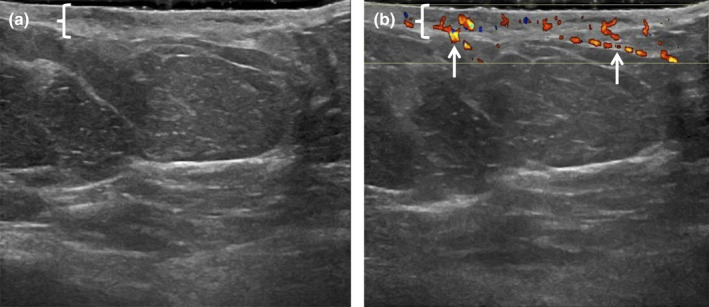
30‐year‐old woman presented with redness and tenderness in the lower central left breast. She was evaluated and started on Bactrim double‐strength twice daily. Subsequently, the patient noticed purulent drainage from the skin with some improvement in her symptoms. The breast ultrasound was performed with a ML6‐15 MHz transducer with a frequency set to 11 MHz. The images were captured from the skin to the chest wall. Targeted ultrasound at the site of focal erythema demonstrated mild skin thickening measuring 4 mm (bracket) with heterogeneous increased echogenicity seen with oedema (a), and associated increased vascularity (b, arrows). No underlying breast findings were observed. Imaging findings are consistent with focal left breast cellulitis without an underlying abscess. Clinical follow‐up of the left breast erythema with repeat targeted imaging in the setting of worsening symptoms was recommended.
